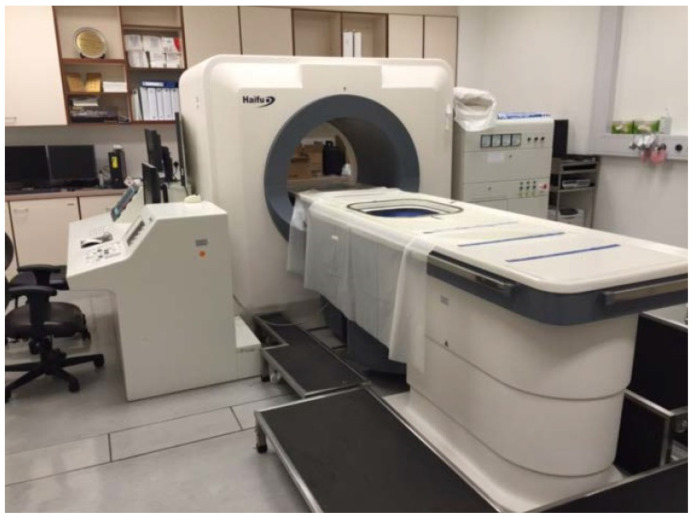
Ultrasound-guided high-intensity focused ultrasound system.
